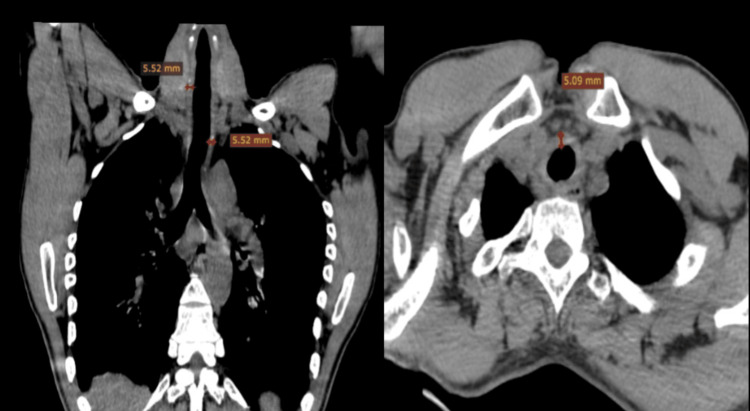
Cervicothoracic computed tomography findings Diffuse, regular, circumferential thickening of the tracheal wall.
